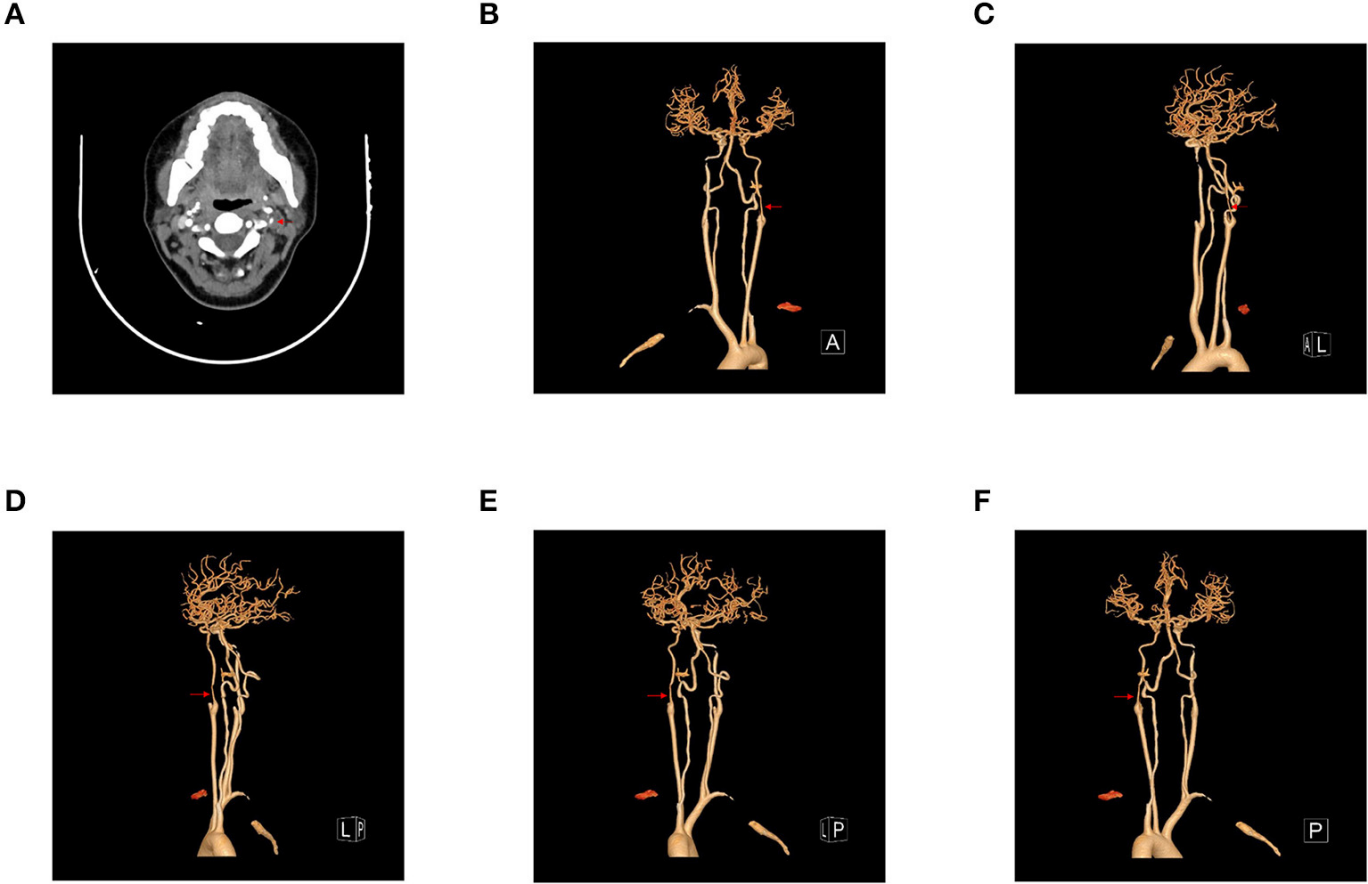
(A–F) Preoperative head and neck computed tomography angiogram (CTA) and three-dimensional reconstruction of head and neck CTA. Head and neck CTA showed dissection and severe stenosis at the origin of the left internal carotid artery.
